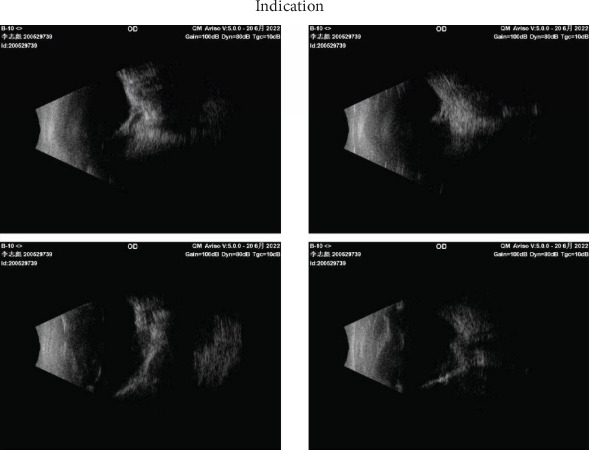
B-scan ultrasound showing a wide range of non-echoic areas behind the eyeball in right eye.
